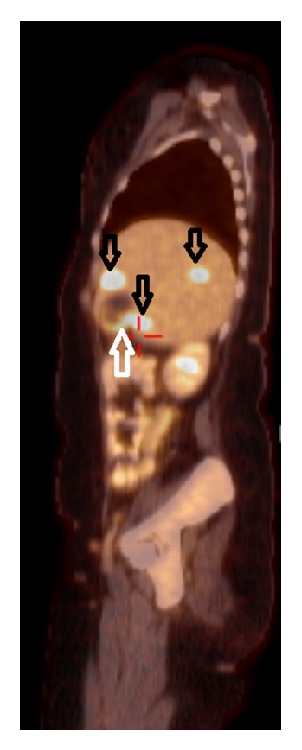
PET-CT showing a hypermetabolic aspect of the uterus (not in this image), gallbladder fundus (white arrow pointing upward), and three liver lesions (black arrows pointing downward), all suspect for neoplastic or inflammatory lesions.
